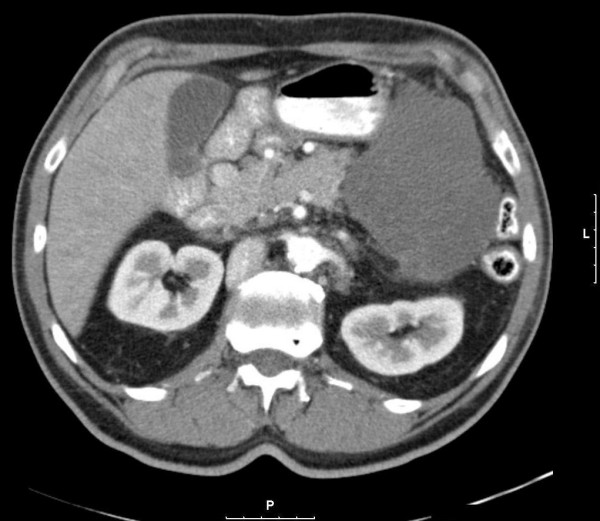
Follow up CT scan after six months of Imatinib. The tumor has shrunk to 10 × 8 cm.
